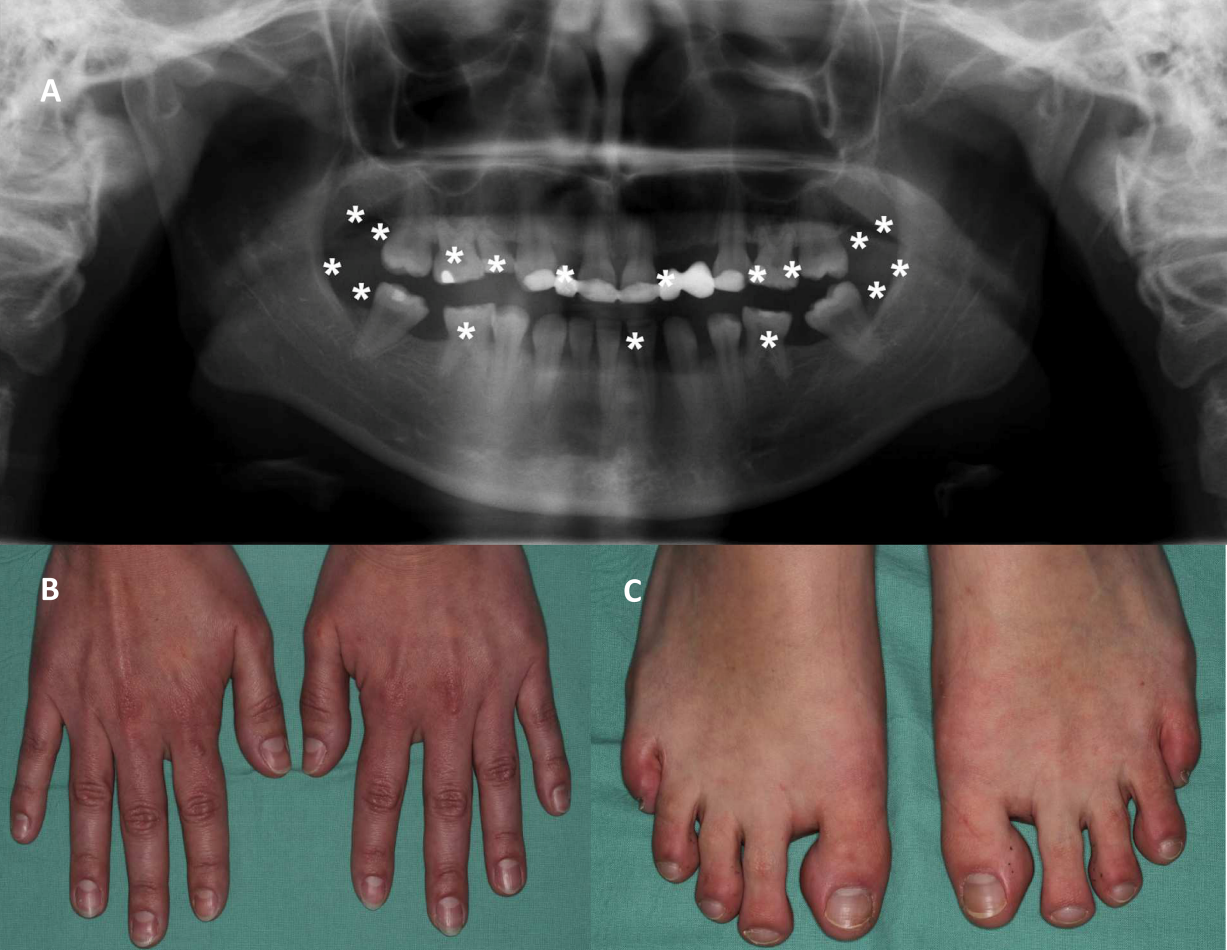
Proband´s tooth and nail phenotype. (A) Panoramic radiograph of proband Z606. This individual has a total 17 congenitally missing teeth (Agenesis 18, 17, 15, 14, 12, 22, 24, 25, 27, 28, 38, 37, 35, 31, 45, 47, 48). Condition after orthodontic and prosthetic treatment. (B) Nails on hands are in normal shape without any defect. (C) Nails on legs are in normal shape and without defects excepts the fifth finger nail that is hypoplastic.
